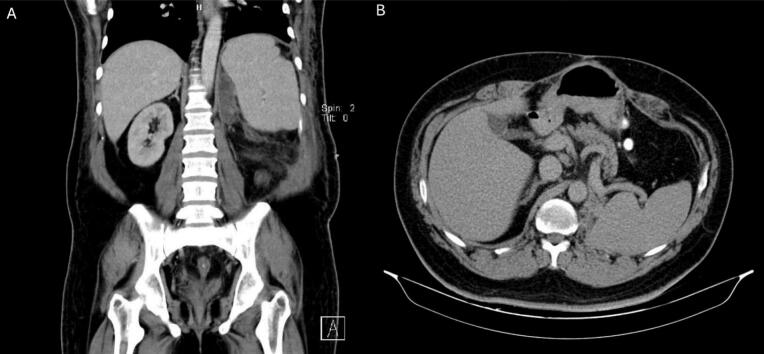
Abdominopelvic CT scan of liposarcoma case 1. A: coronal view displaying the extent of the liposarcoma in the vertical plane encasing the left kidney. B: axial view showing the transverse cross-section of the liposarcoma extending to the retroperitoneal area.
